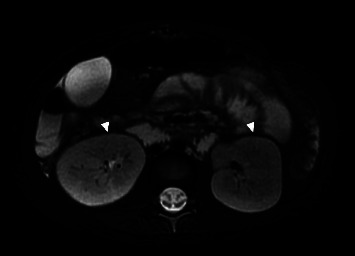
Axial T2 weighted MRI image without IV contrast shows borderline enlarged bilateral kidneys with lack of corticomedullary differentiation (arrowheads), suggesting some mild degree of autosomal recessive polycystic kidney disease.
